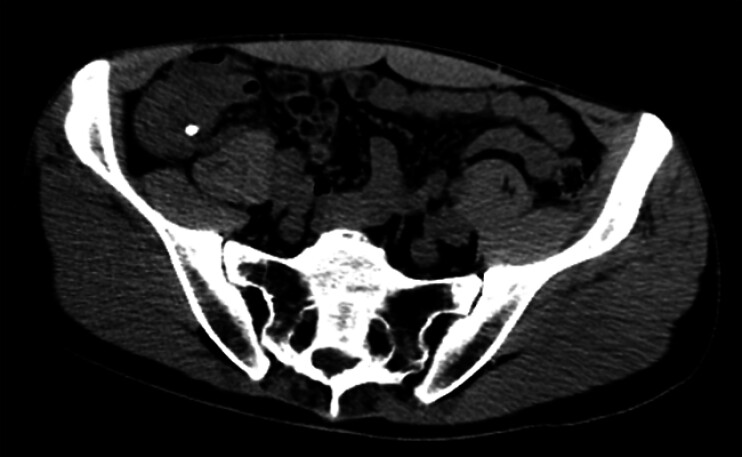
Computed tomography scan of the pelvis showing an appendiceal fecal stone with evidence of chronic appendicitis.
